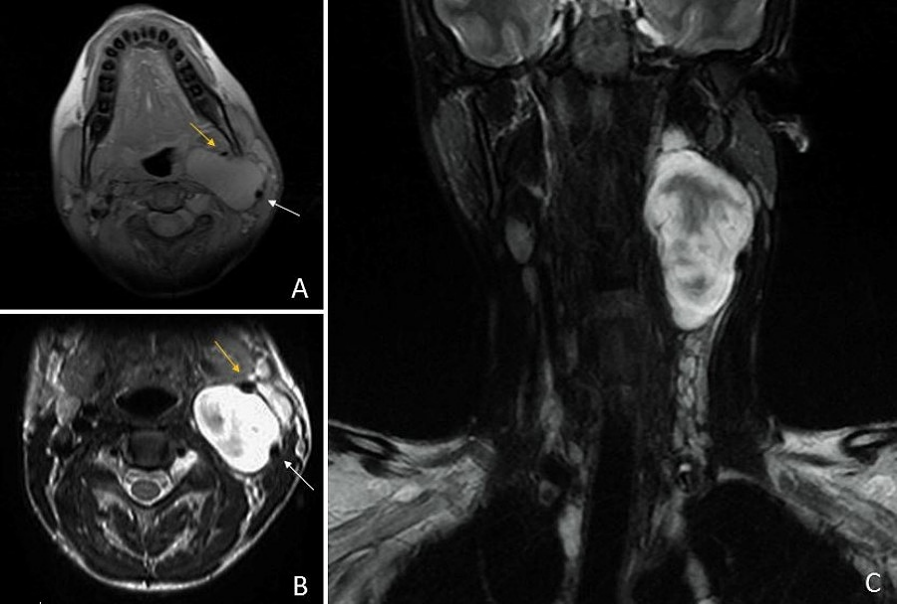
Preoperative axial and coronal cervical spine magnetic resonance imaging (MRI) Preoperative cervical MRI showing a large mass at the left carotid bifurcation with splaying of the left internal carotid artery (white arrow) and external carotid artery (yellow arrow). A) T1-weighted, axial, isointense; B) T2-weighted, axial, predominantly hyperintense; C) T2-weighted, coronal, predominantly hyperintense
